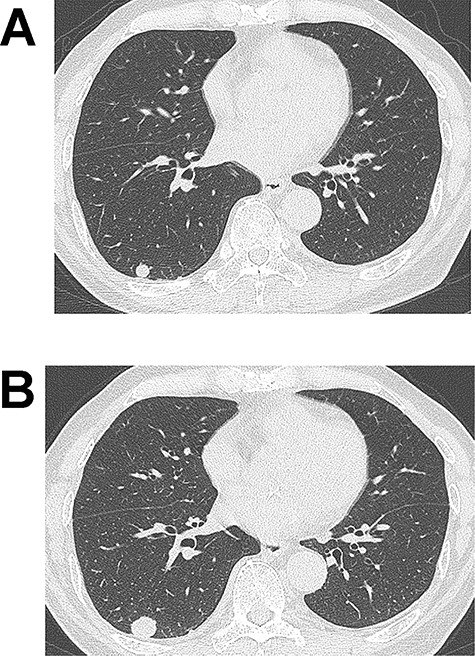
(A) Computed tomography (CT) image showing a 10-mm nodule in the lower lobe of the right lung. (B) The tumour grew to 17 mm in diameter on CT 2 years after the initial imaging.
